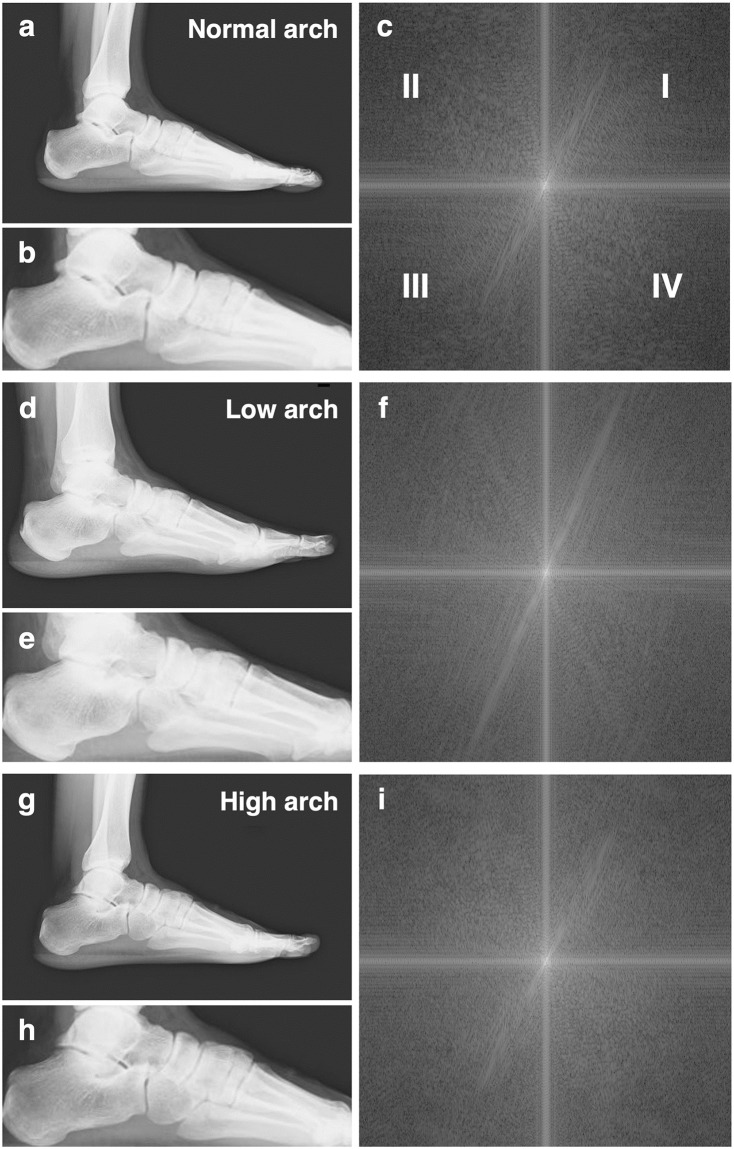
The texture analysis of lateral foot bone. The analysis process for a normal arch foot (a-c), a low arch foot (d-f), and a high arch foot (g-i) in each example is shown below. The original x-ray radiographs (a, d, and g) were leveled. Then ROIs were set up with the upper surface of the talus pulley as the upper edge, the bottom from the calcaneus base to the metatarsal head base, and the right and left edges to the calcaneal prominence and metatarsal head (b, e, and h). The 2D-FFTs in these ROIs were processed using ImageJ2 (c, f, and i). Roman numerals I, II, III, and IV indicate the respective quadrants.
